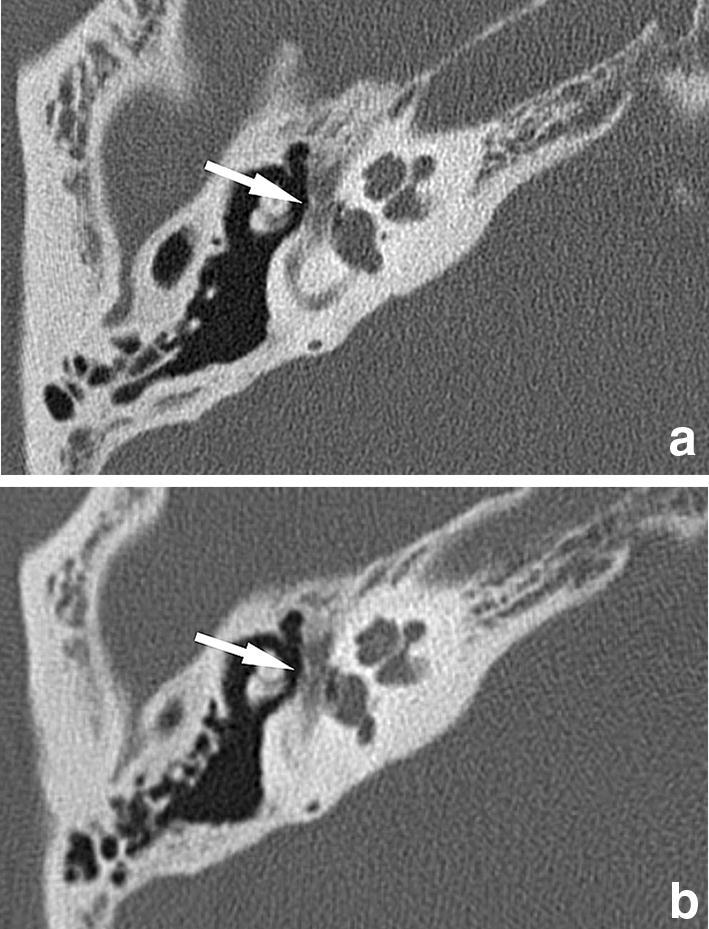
Axial images of the right temporal bone CT from the same case. Image quality scores for the tympanic segment of the facial nerve canal (arrow) by Reviewers 1 and 2 are three and four on UHRCT (a), respectively, and two and three on MDCT (b). Dehiscence in this anatomy was considered to be present by both Reviewers on MDCT; only by Reviewer 1 but not by Reviewer 2 on UHRCT
